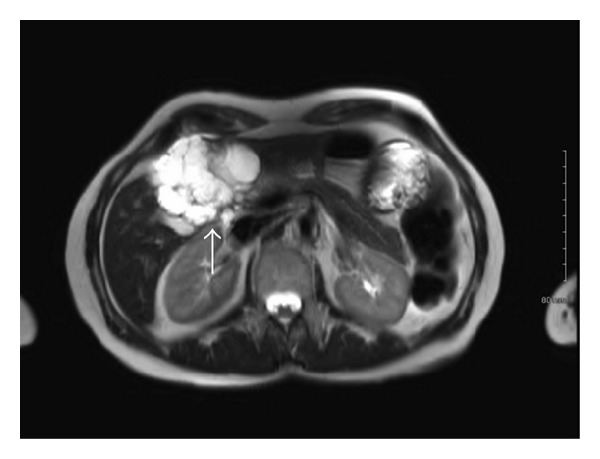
MRI image detailing a 6.6 × 5 cm cystic mass originating from segment 4B of the liver (arrow).
